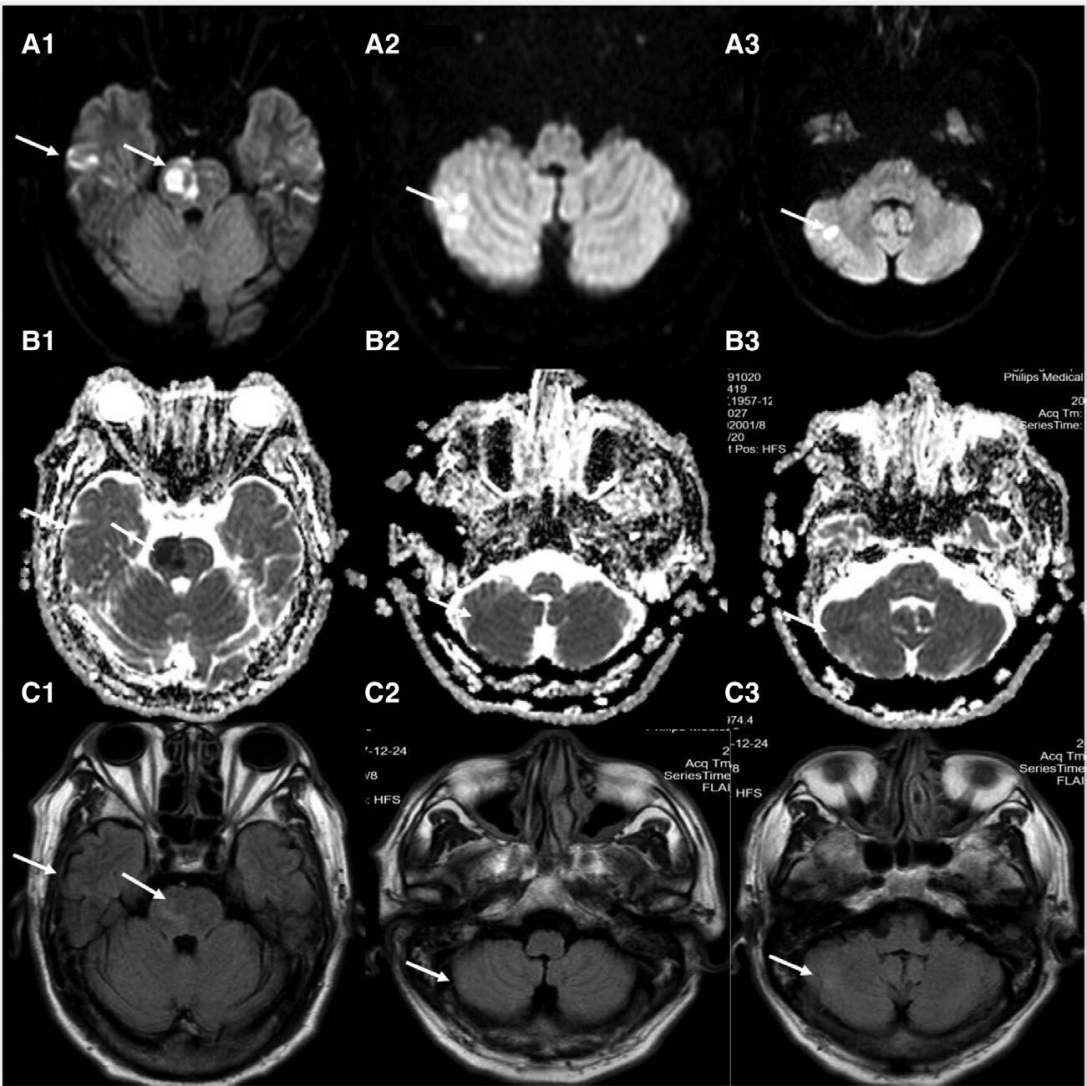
On admission, magnetic resonance imaging of the head showed recent multiple infarcts in the brainstem and right hemisphere. (A1–A3) Represent the DWI sequence. (B1–B3) Represent the apparent diffusion coefficient sequence, and (C1–C3) represent the T2 Flair sequence. The white arrows indicate the locations of the infarcts.
